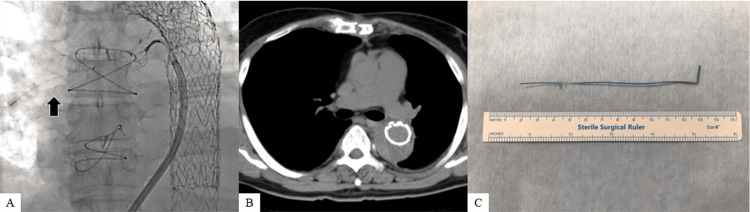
Catheter therapy to remove the TEPW. (A) Intraoperative finding (Black arrow: residual TEPW in the right pulmonary artery). (B) Computed tomography after catheter therapy. There is no residual wire in the right pulmonary artery. (C) Removed residual TEPW. TEPW: Temporary epicardial pacing wire
